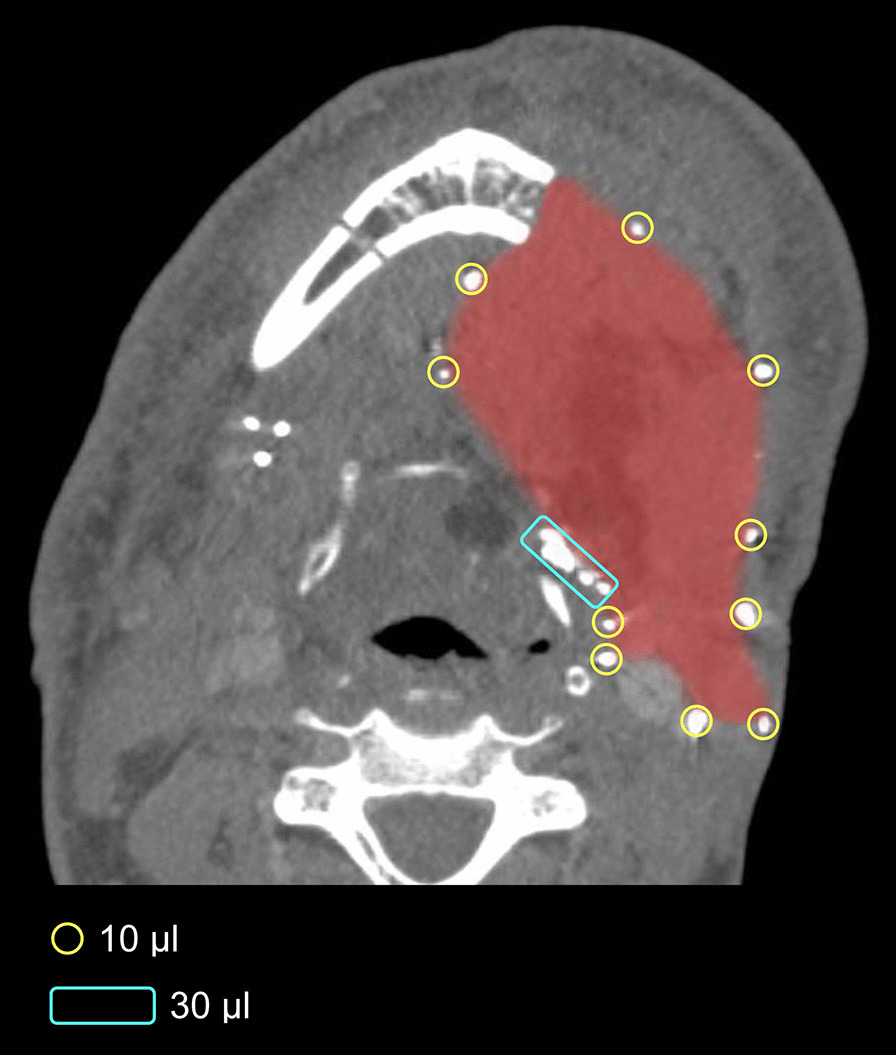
Markers created by injection of 10 µl of SAIB/x-SAIB guide delineation of the tumor resection surface in postoperative CT imaging. Moreover, markers created by the injection of 30 µl of SAIB/x-SAIB can be visually differentiated from the 10 µl markers and thus be applied to mark regions which require special attention in the postoperative setting
